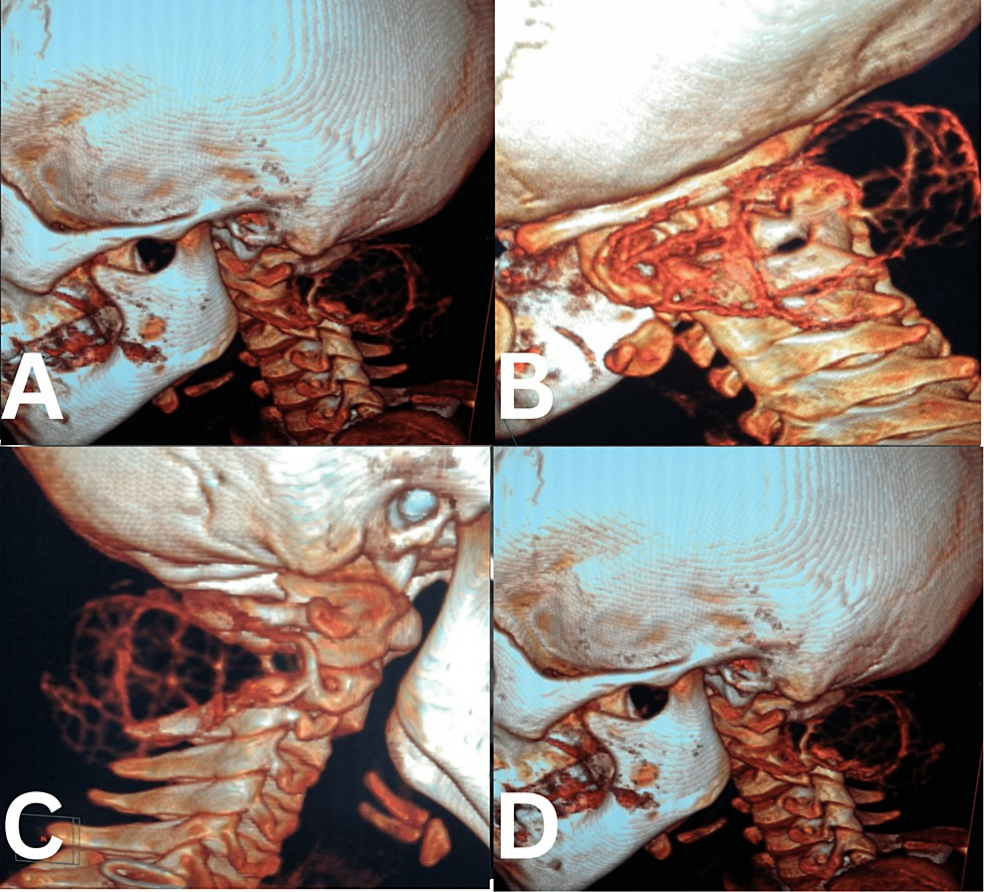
Neck CT scan three-dimensional images. (A, B) sagittal; (C) oblique; (D) coronal sections.
